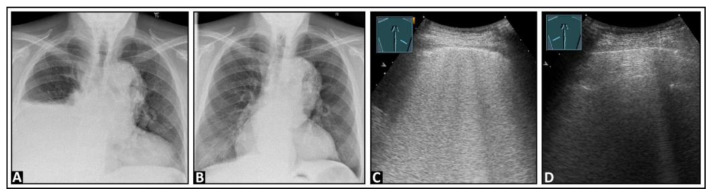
A patient with dyspnea and right-sided pleural effusion shown on chest X-ray images before (A) and after (B) an effusion-relieving puncture. (C) After puncture, on lung ultrasound, multiple confluent B-lines appear on the right side, suggesting a “white lung” pattern, as seen in re-expansion edema; (D) the left lung shows an inconspicuous pleural reflection band.
